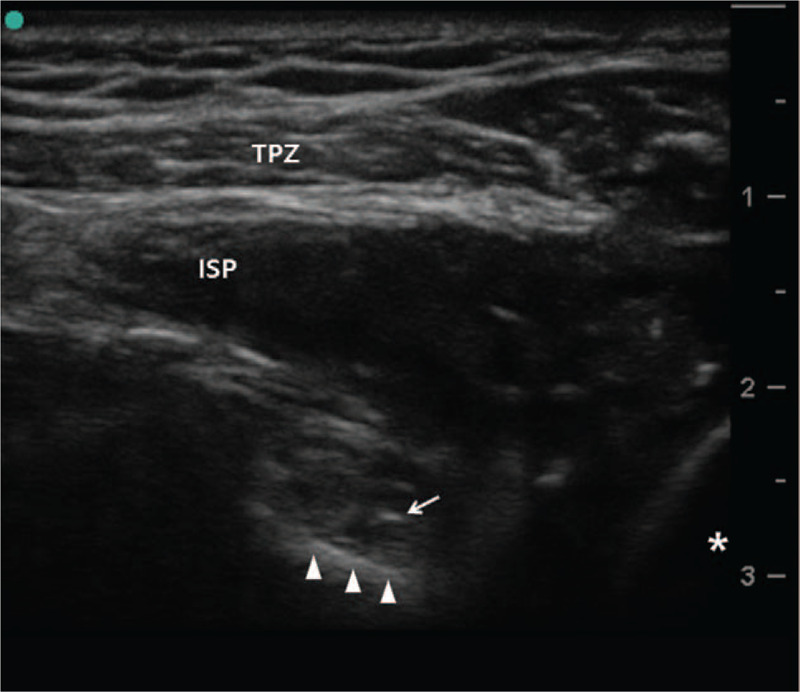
Ultrasound imaging of applying radiofrequency probe on suprascapular nerve in the spinoglenoid notch. Arrow indicates the needle tip and arrow heads indicate spinoglenoid notch near the glenoid (asterisk). ISP = infraspinatus muscle, TPZ = trapezius muscle.
